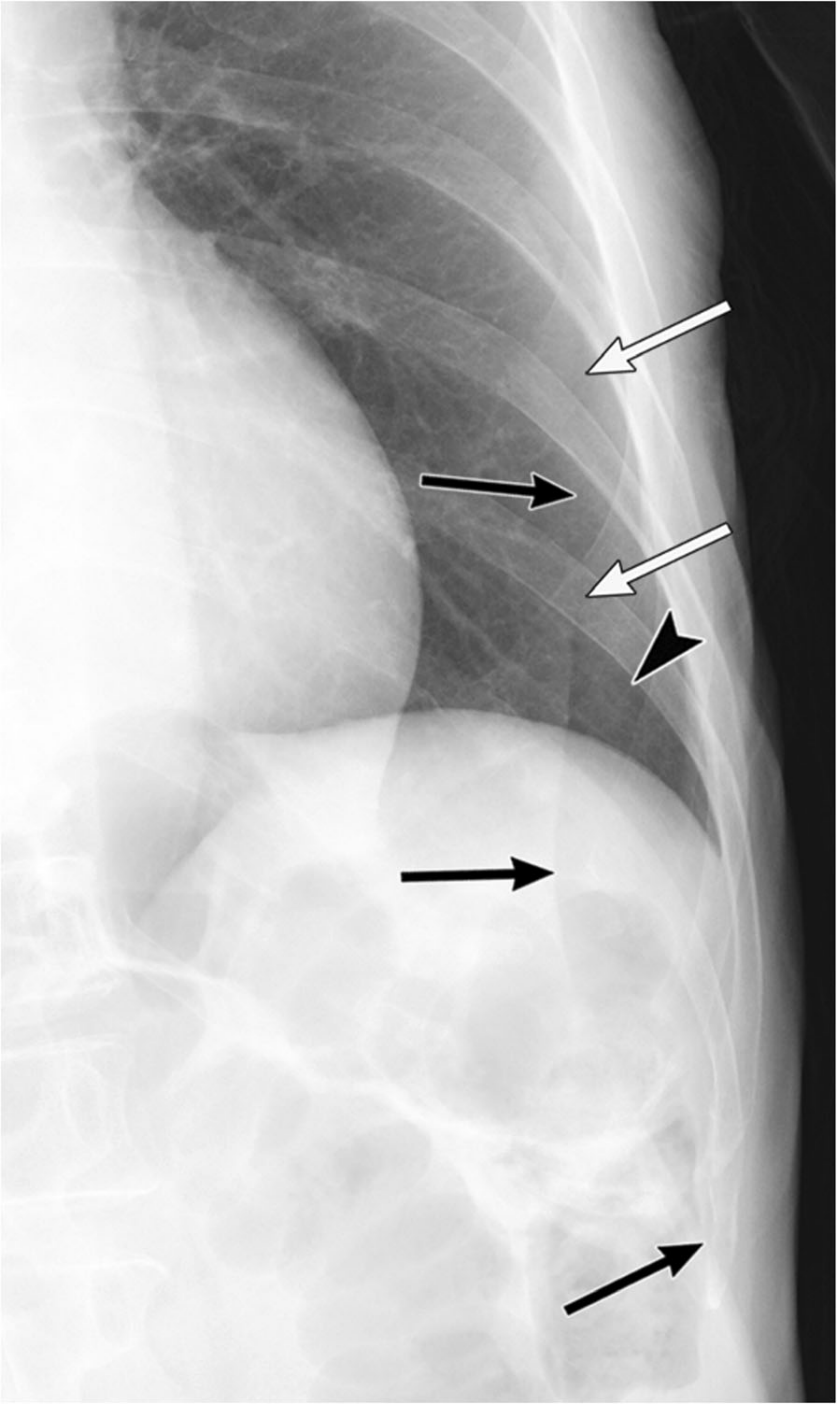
A skin fold mimicking pneumothorax on a radiograph in a 66-year-old woman with glioblastoma. The skin fold appears as a broad radiopaque band (black arrows) that is adjacent to a radiolucent band (white arrows), which reflects a negative Mach band effect in the left lung field on a supine radiograph. It extends beyond the pleural cavity. Vascular markings (black arrowhead) are visible beyond its edge
